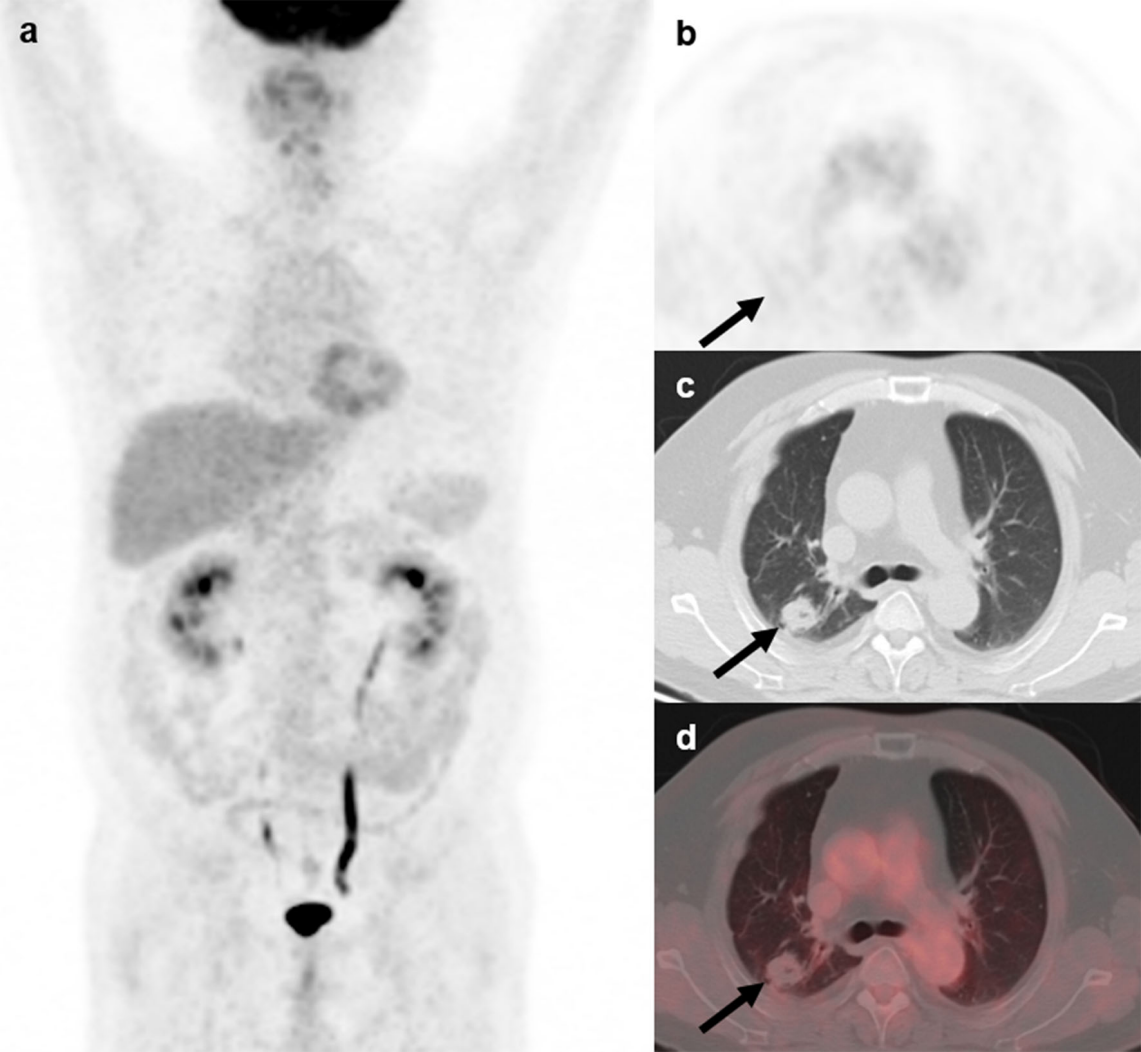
^18^F-FDG PET/CT findings in a representative case of aspergillosis (Patient, 4). A 39-year-old man diagnosed with ectopic Cushing syndrome underwent ^18^F-FDG PET/CT to locate the possible ACTH-secreting tumor. ^18^F-FDG PET/CT (A) showed a nodule in the right lung demonstrating slightly higher-than-background activity (B–D; arrows; SUV_max_, 1.0). Subsequent percutaneous lung biopsy confirmed the diagnosis of pulmonary aspergilloma.
